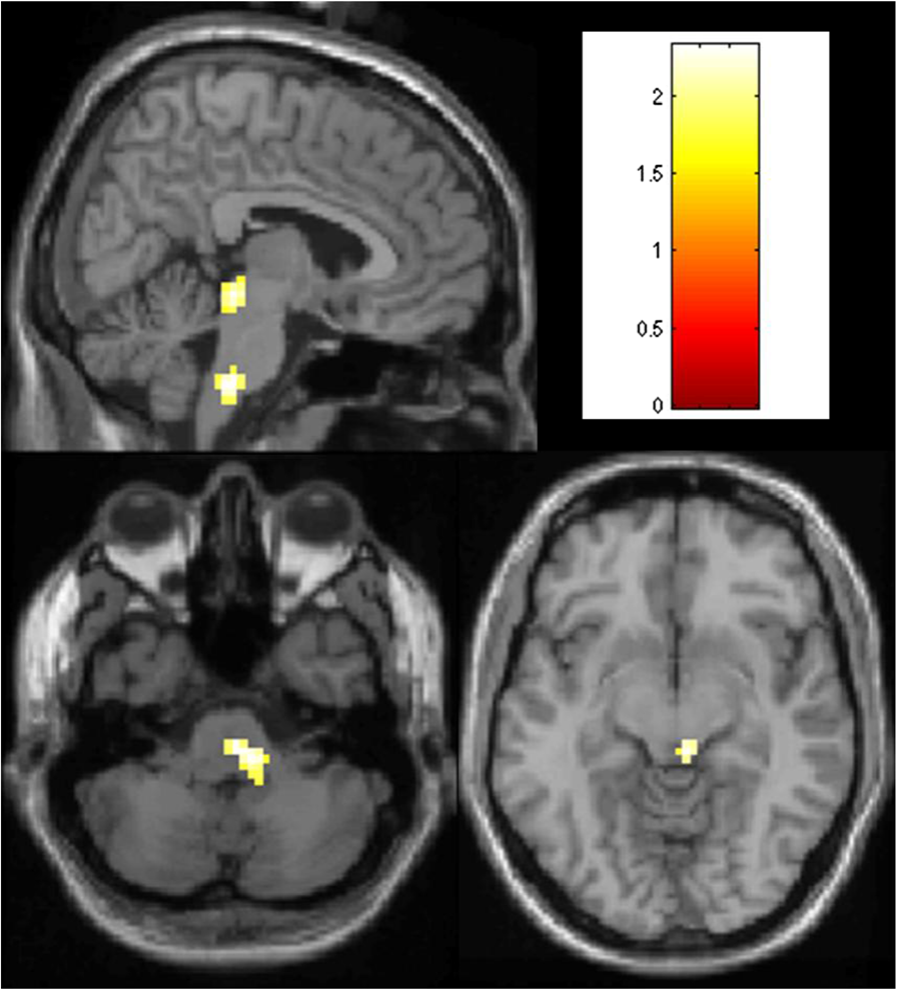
Areas of increased rCBF in the premonitory phase of migraine in patients with nausea > patients without nausea. Areas of increased rCBF in the premonitory phase > baseline are depicted in patients with nausea > patients without nausea. The results are superimposed on an anatomical reference derived from a representative T1-weighted MRI of one of the patients in this study. The colour bar indicates the colour coding for the Z scores. Images are displayed in radiological convention i.e. left side of image is right side of brain. A threshold of *p* < 0.01, uncorrected, was used for display purposes.
